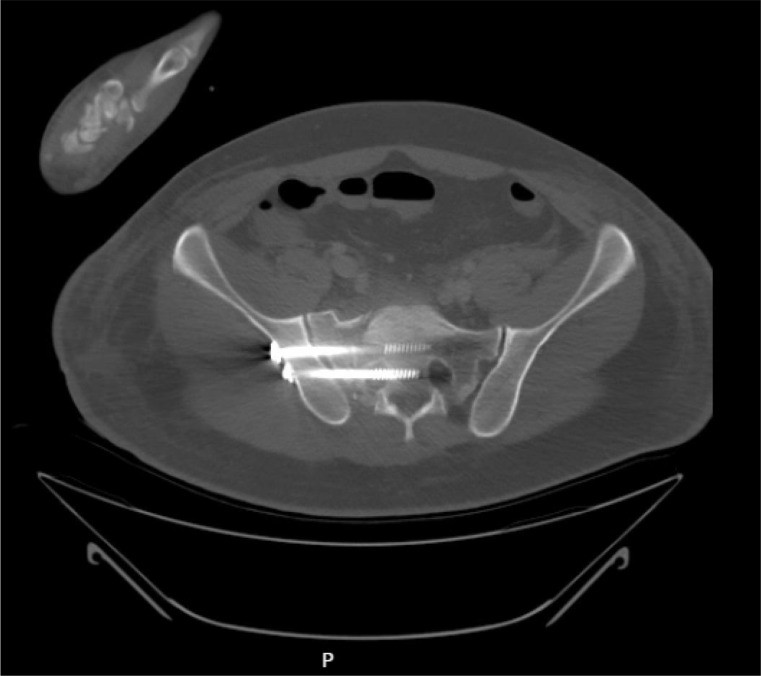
Example of a malpositioned SI screw. Axial view of CT scan. The posterior SI screw traverses the right neural foramen
